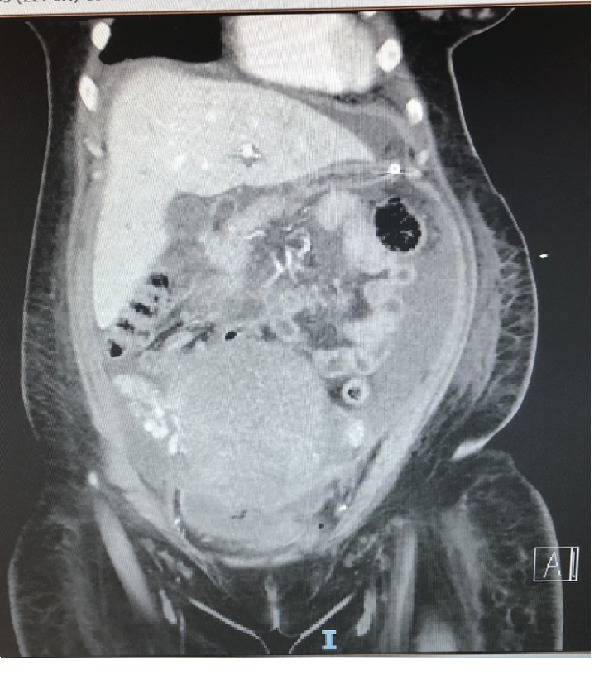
CT abdomen and pelvis showing ascites out of keeping with recent caesarean section.
